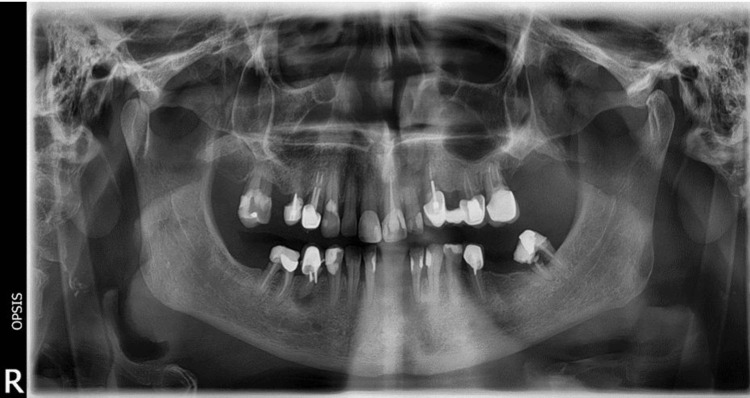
Preoperative Orthopantomograph
